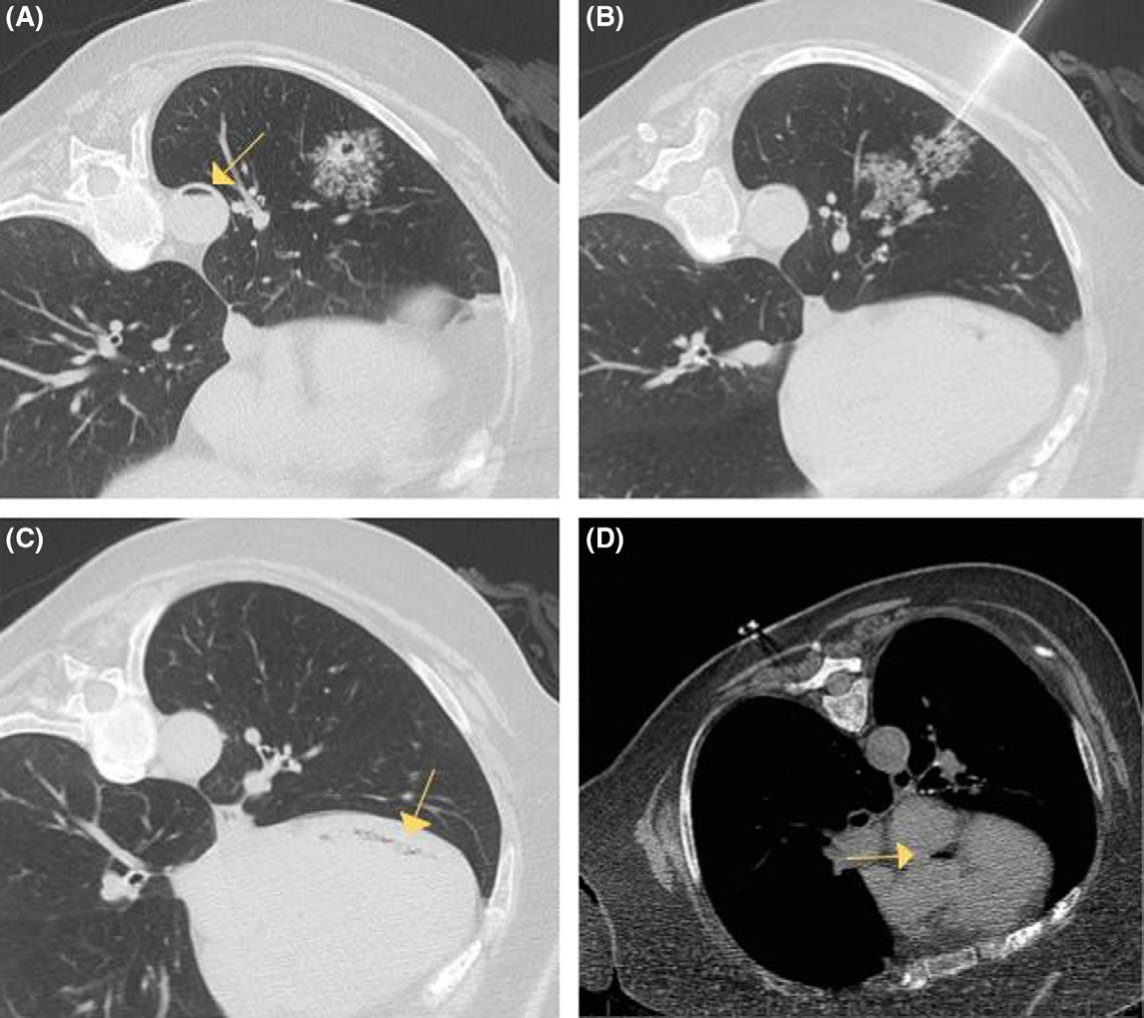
Computed tomography (CT) during biopsy demonstrating multiple areas of intravascular air
